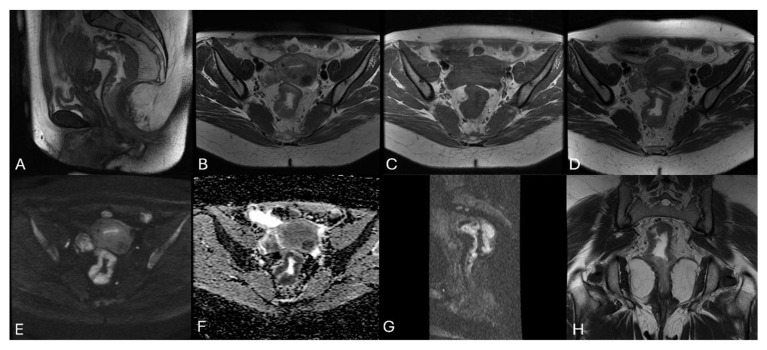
Representative images of each MRI sequence acquired for staging. (A) Sagittal 3D T2w; (B) Axial fast recovery FSE (FRFSE) T2w; (C) Axial T1w; (D) Oblique axial T2w; (E) Axial DWI (b-value = 1000); (F) Apparent diffusion coefficient (ADC) map; (G) Oblique coronal T2w. (H) Oblique coronal T2w.
